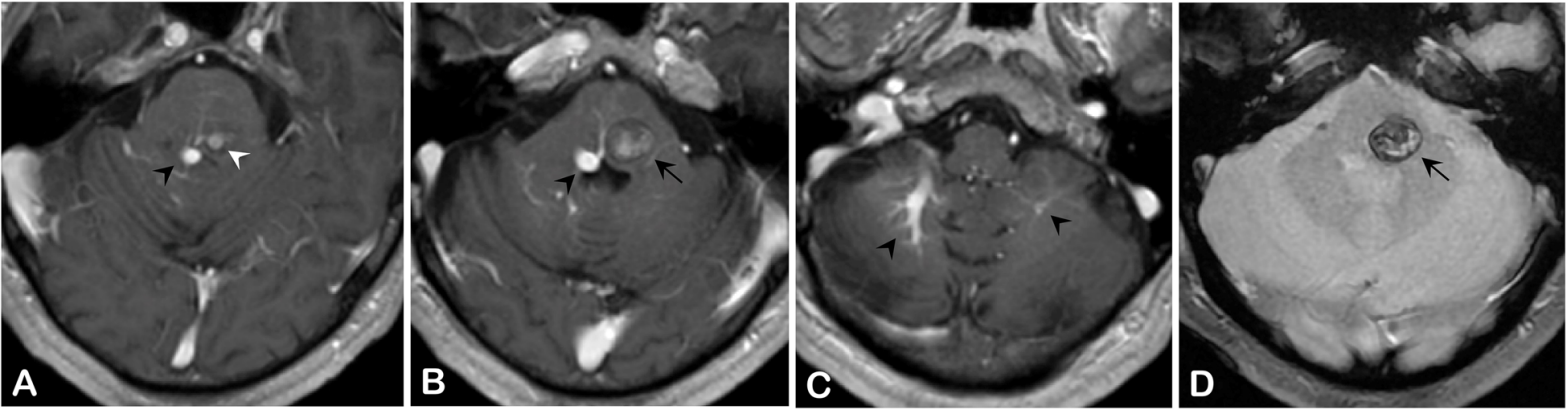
The cerebral MRI shows (a, white arrowhead, post-contrast T1-weighted sequence) a brainstem cavernous malformation (BCM) associated with (a,b,c, black arrowheads, post-contrast T1-weighted sequence) a bilateral cerebellar developmental venous anomaly (DVA) prevailing on the right side and draining into the vein of Galen. (b, black arrow, post-contrast T1-weighted sequence and D, back arrow, gradient-echo sequence) The BCM was responsible for a medial pontine hematoma
